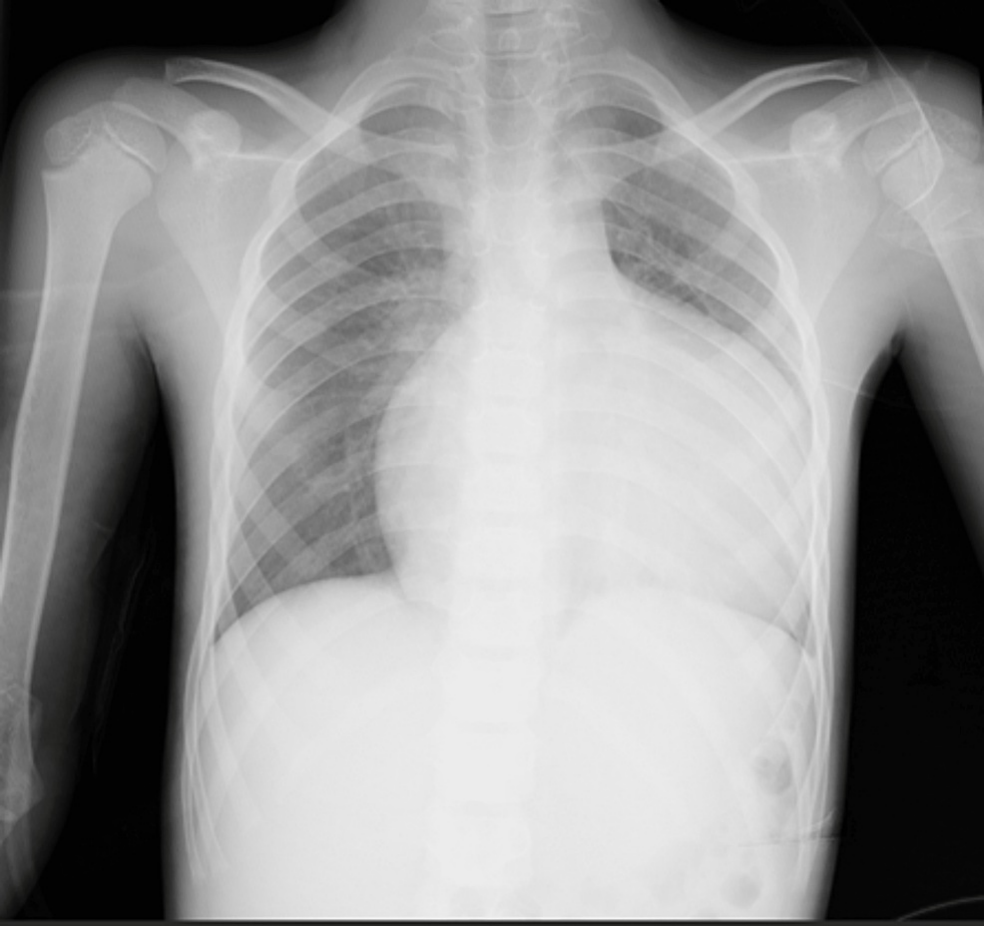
Chest X-ray showing massive cardiomegaly and hilar congestion
